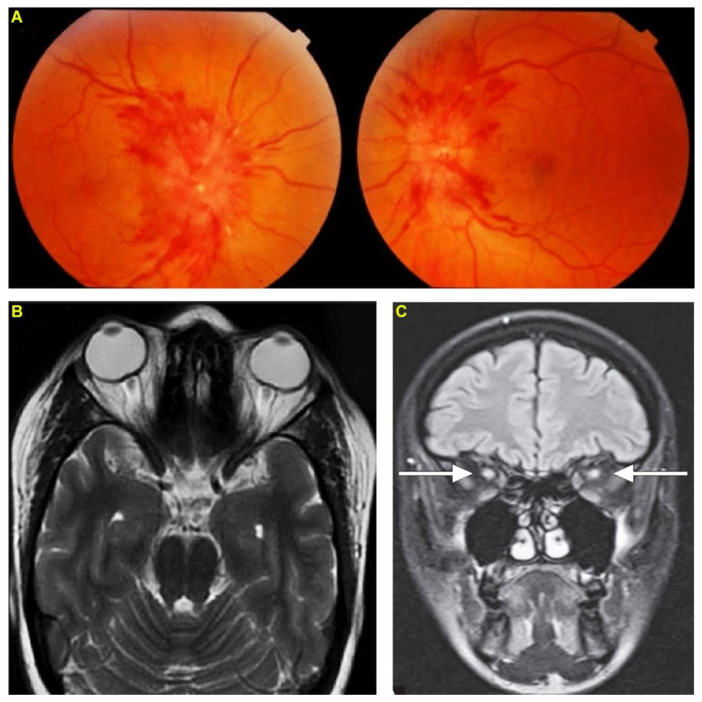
Envenomation by an Indian cobra induces optic neuritis. Patient 1 following a bite from an Indian cobra has developed bilateral papilledema (A). (B) magnetic resonance imaging orbit shows effacement of the perioptic-optic cerebrospinal fluid space and diffuse increase in T2 signal involving the optic nerve. (C) coronal fat-suppressed T2-weighted magnetic resonance imaging shows hypertense thickening of perioptic nerve sheath surrounded by cerebrospinal fluid providing a ‘polo-mint’ appearance (indicated by arrows).
